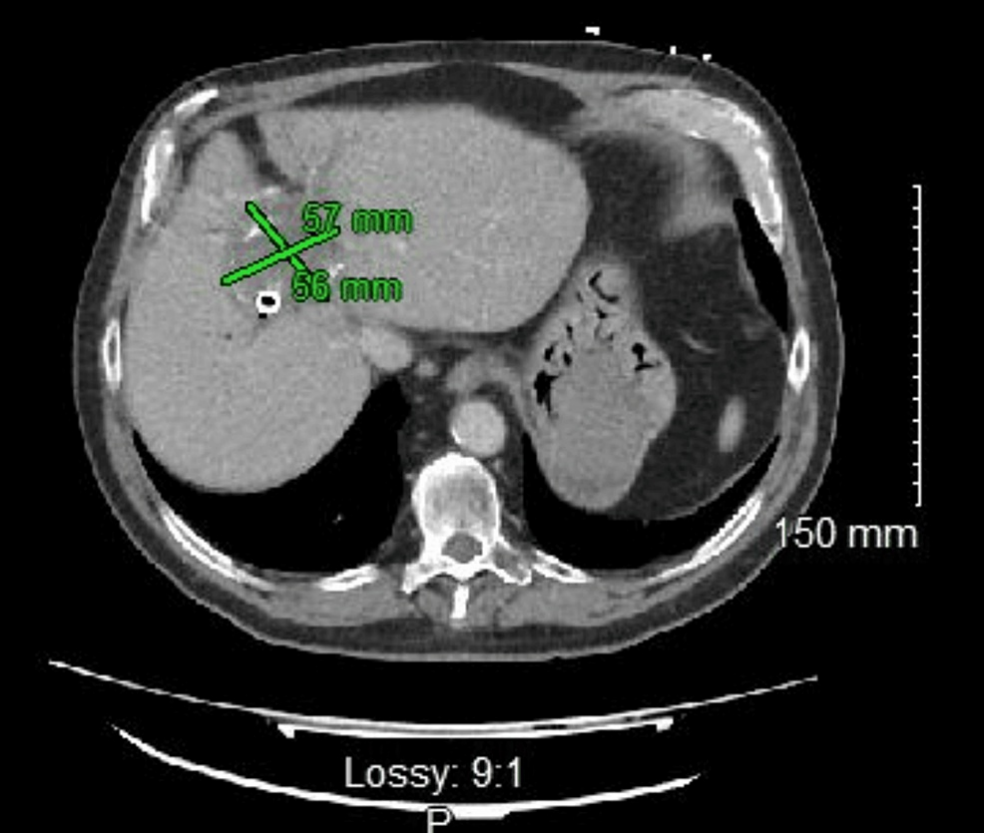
Axial CT image of the upper abdomen. There is diffuse hemobilia (green cross mark). The green cross mark measures the size of hemobilia within the liver.
